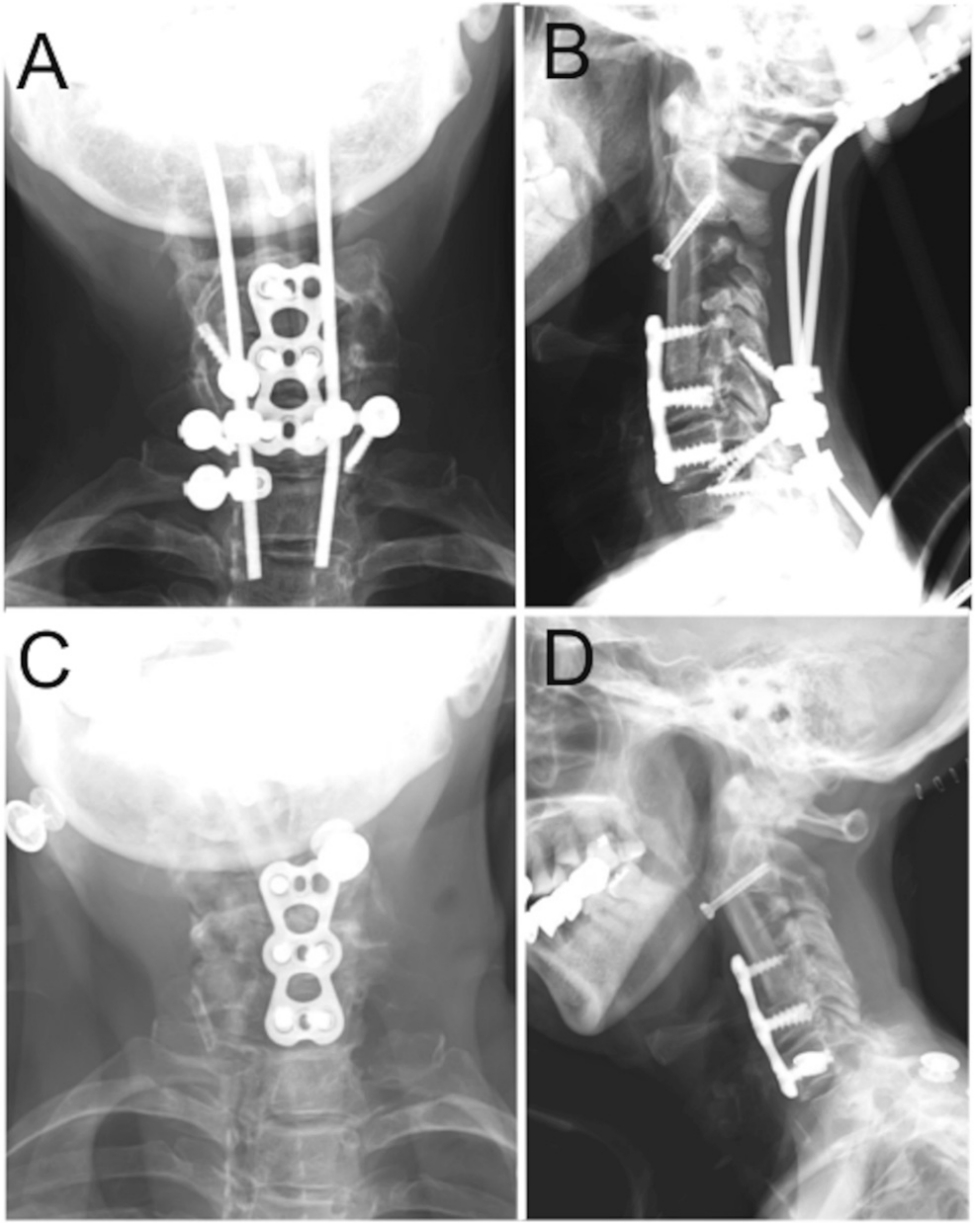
X-ray Images Following Instrumentation Failure Due to instability, the left Th1 pedicle screw is removed (A, B). Fusion does not occur between the fibula graft and C2 vertebral body, leading to the displacement of the canulated mini screw (C, D).
